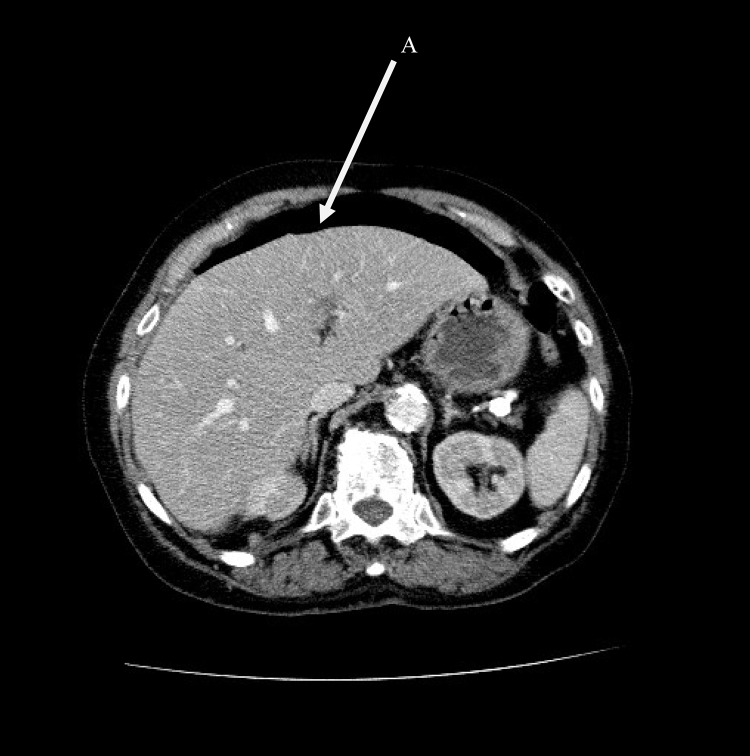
Axial computed tomography showing free intraperitoneal air (A) in the upper abdomen, suggestive of gastrointestinal perforation
